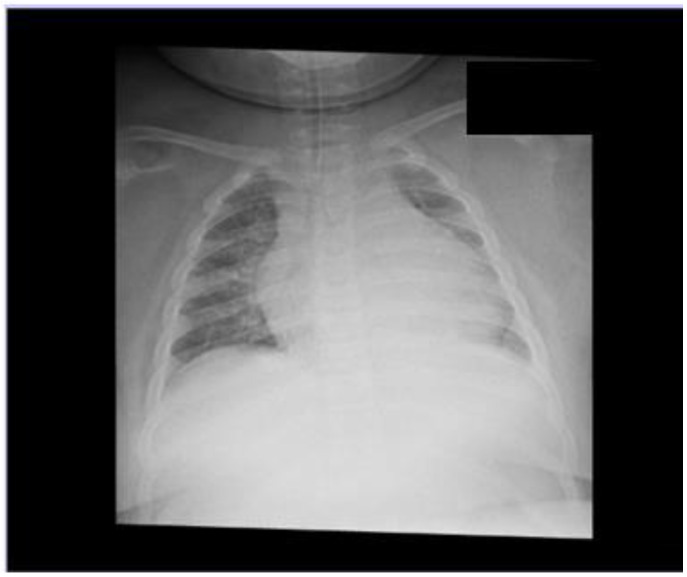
Bedside chest x-ray in supine position. Left lung obscured by the shadow of a greatly enlarged heart.
